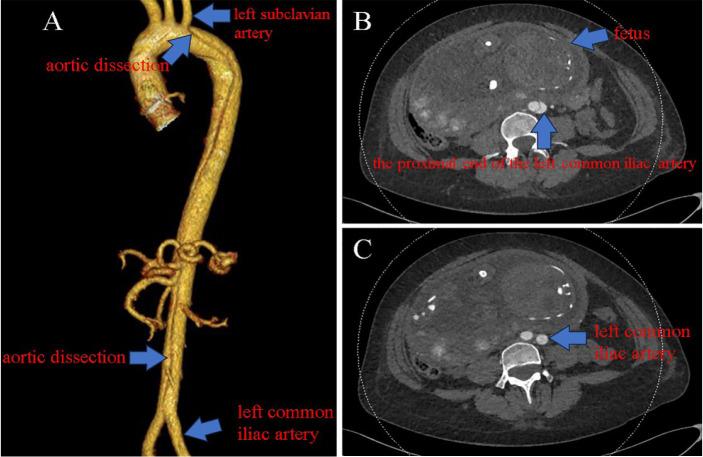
Computed tomography angiography images. A) The aortic dissection starts
